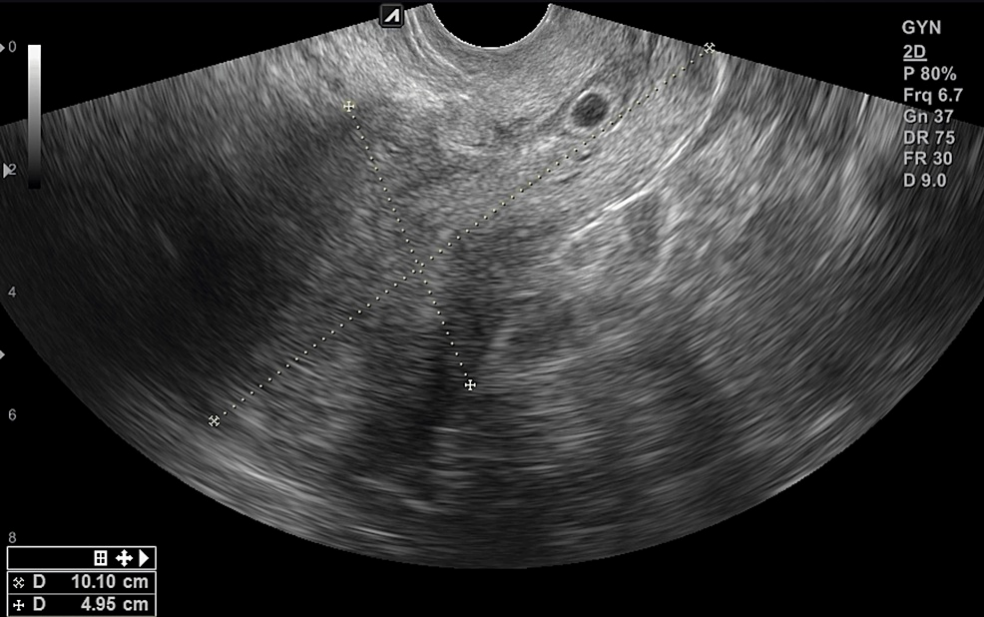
Transvaginal ultrasound performed at the initial evaluation shows an anechoic structure with a poorly delimited internal ring observed within the posterior lip of the cervix.
